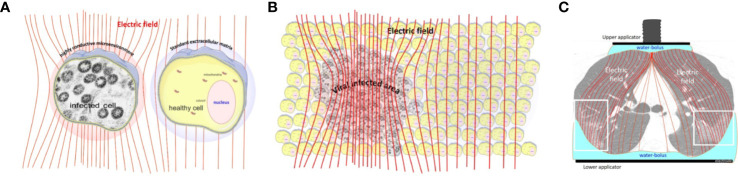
The radiofrequency current flows into the direction of the lower impedance. (A) The increased metabolic activity of the infected cells allow lower impedance (higher conductance) in their microenvironment than in the healthy cells. (B) The electric field selects the volumes with a high concentration of the infected cells. (C) the lung-lesions are loaded more with the radiofrequency current than the non-infected parts [The CT picture is from (74)].
